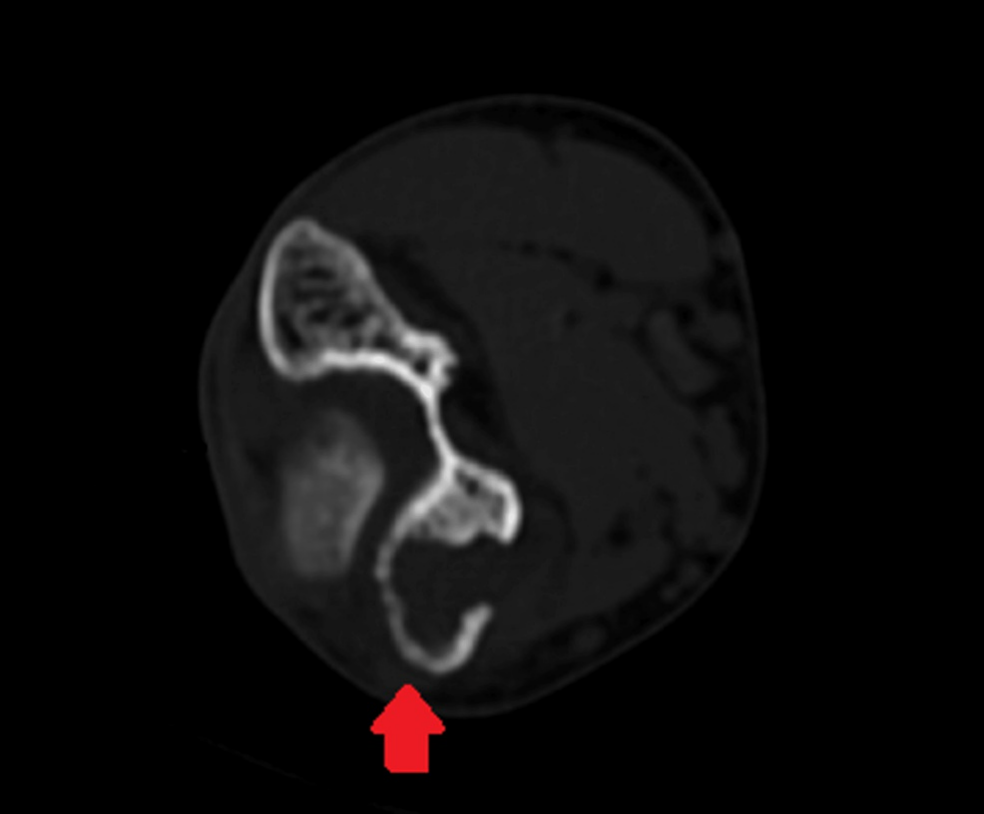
CT scan of the left elbow joint Arrow showing a lytic lesion involving the medial epicondyle of the humerus, cortical erosions, and adjacent soft tissue extension CT: computed tomography
